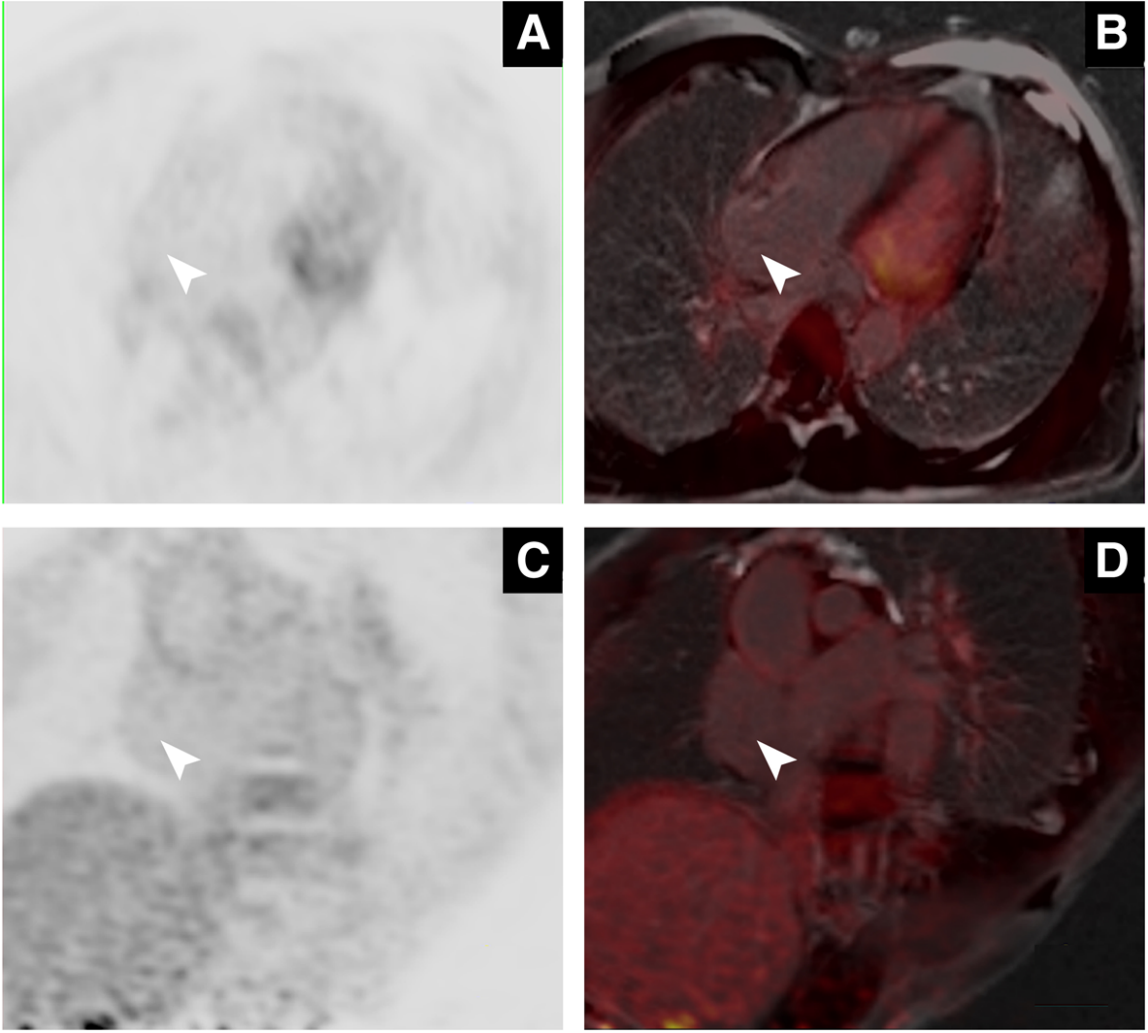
The four chamber view of the heart using PET (a) and fused PET/MRI (b) demonstrated no obvious radiotracer uptake on the mass (arrowheads). Short axis view of the heart using PET (c) and fused PET/MRI (d) also indicated nothing unusual on the mass (arrowheads)
